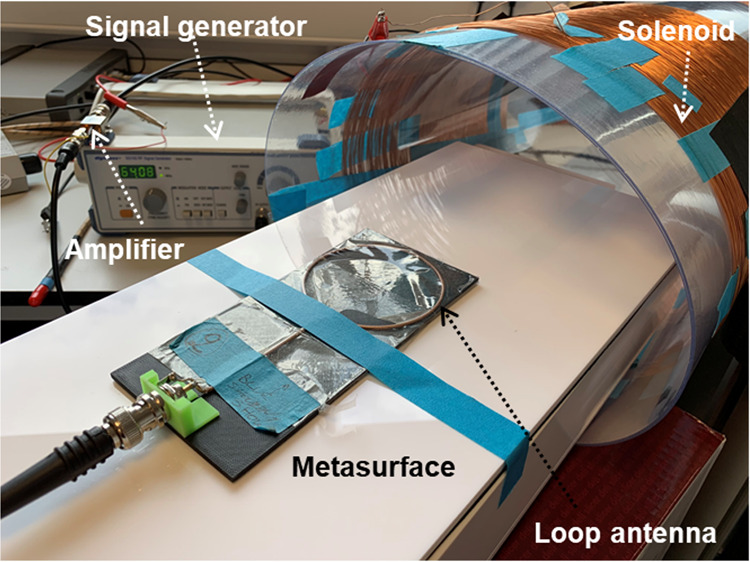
The solenoid made from insulated copper wire to mimic the MRI RF magnetic field for automatic detection. The solenoid is fed by an RF signal generator followed by an 18 dB gain amplifier. A broad band loop coil is used to measure the resonance frequency^23,24^.
